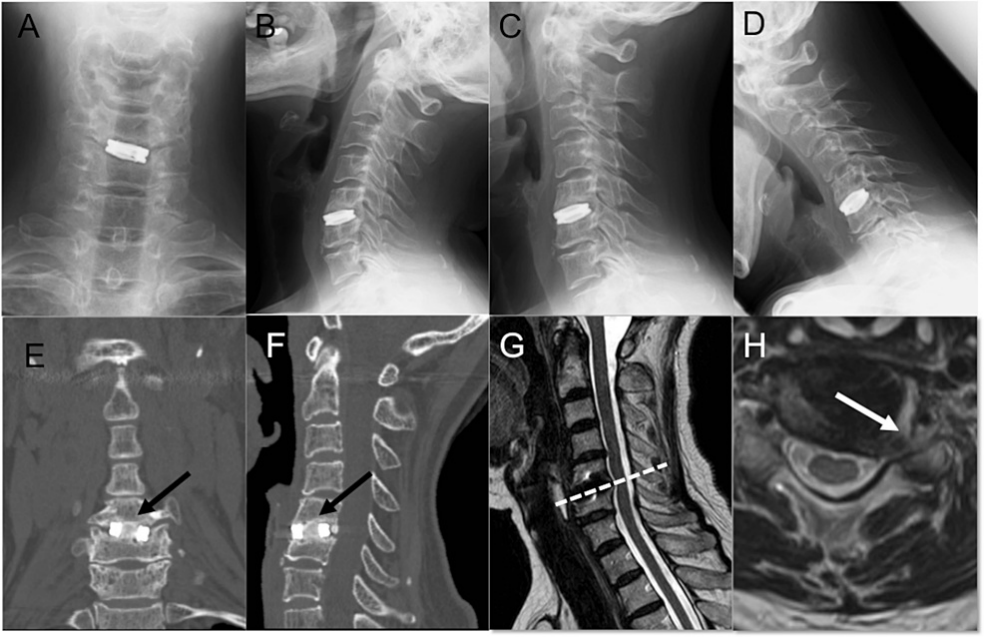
Preoperative radiological findings of Case 1 A titanium spacer was observed at the C5/C6 level. Instability was observed at the C4/C5 level in the flexed position. Instability at the C5/C6 level was not apparent (A: anteroposterior X-ray image, B-D: lateral X-ray images). Calcification in the titanium cage was considered in computed tomography images. The signal intensity of the calcification in the titanium cage was different from that in the vertebral bones (black arrows) (E: axial image, F: sagittal image). The left nerve root of C6 was compressed owing to the presence of osteophytes (G and H: magnetic resonance images).
